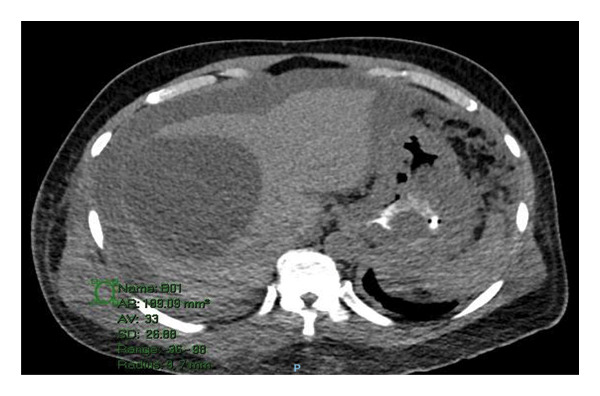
CT scan finding of a thin‐walled fluid collection is better seen in the region of the right upper paraspinal and trapezius muscles having a dimension of about 3.3 × 7.4 × 3.7 cm (AP × T × CC). Some hyperdense components are also seen. Minimal fluid collection is also noted underneath the right serratus anterior. Subcutaneous and myofascial edema are noted in both sides of the chest, but mostly on the right.
